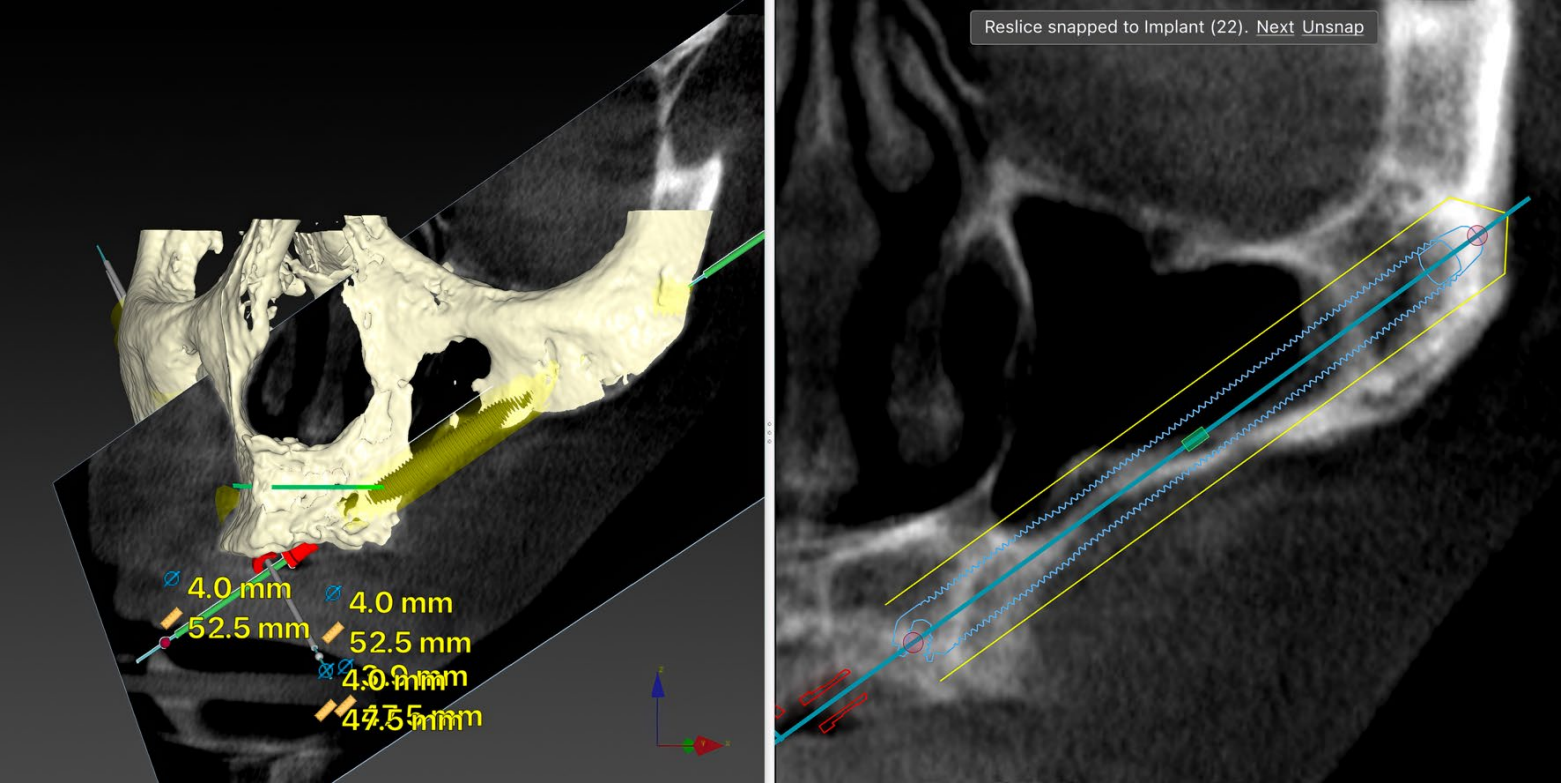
The oblique CBCT slice, taken using DTX Studio Implant software, shows the planning and anatomy at the level of the left lateral incisor of the ZAGA Round implant in Fig. 1. Although the patient moved during the acquisition, we declined to perform another CBCT
